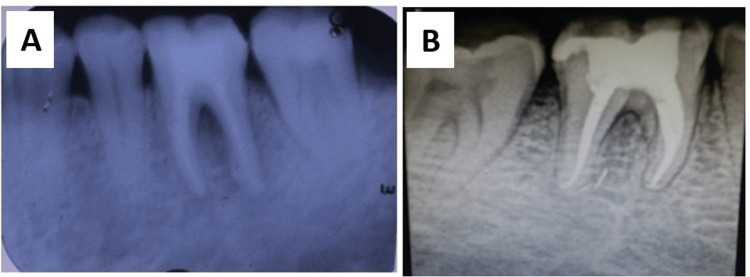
Preoperative (A) and postoperative (B) radiograph at the third month follow-up
